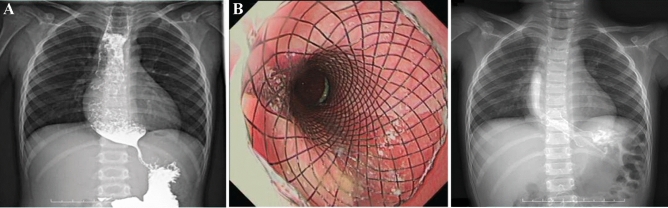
Stent placement in a patient with achalasia of cardia (case 12). (A) The upper gastrointestinal tract radiography showed a beak-like change, suggesting it was difficult for the barium to pass through before surgery; (B) A stent was placed and expanded as the patient body temperature rose; (C) Upper gastrointestinal tract radiography showed normal passing through of the contrast agent after stent placement.
